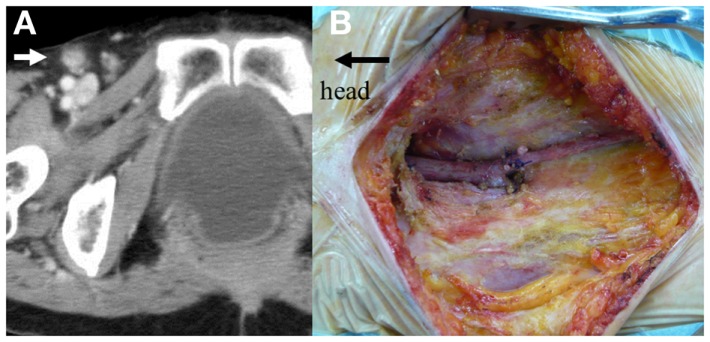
(A) Pelvic computed tomography reveals swelling of the right inguinal lymph nodes (white arrow). (B) Intraoperative photograph after right inguinal lymphadenectomy.
